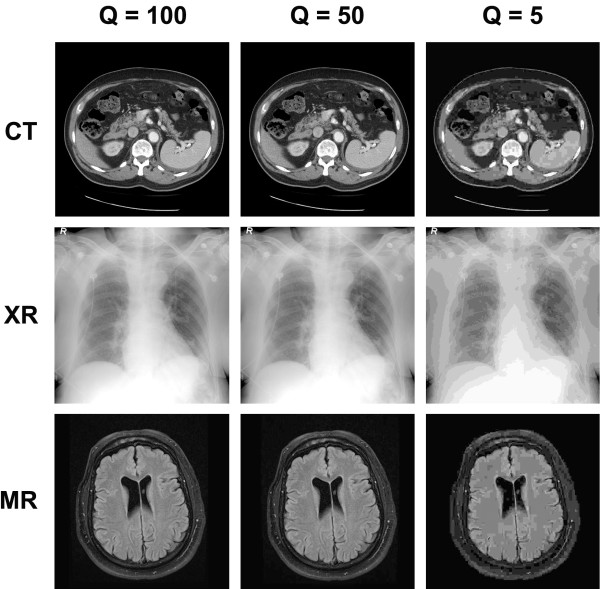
Example images: JPEG compression. An example of each of the imaging modalities studied (CT, XR, and MR) is shown at three levels of JPEG Q value (100, 50, and 5).
